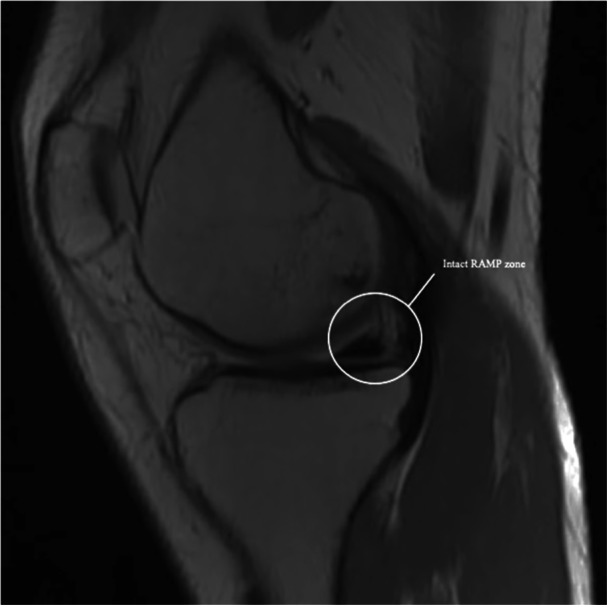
Magnetic resonance imaging (MRI) view: negative for RAMP lesion.
